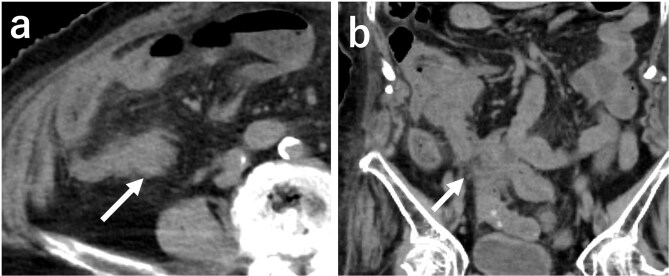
CT 28 days before mastectomy. (a–b) Mild fluid collection can be seen around the appendix.
